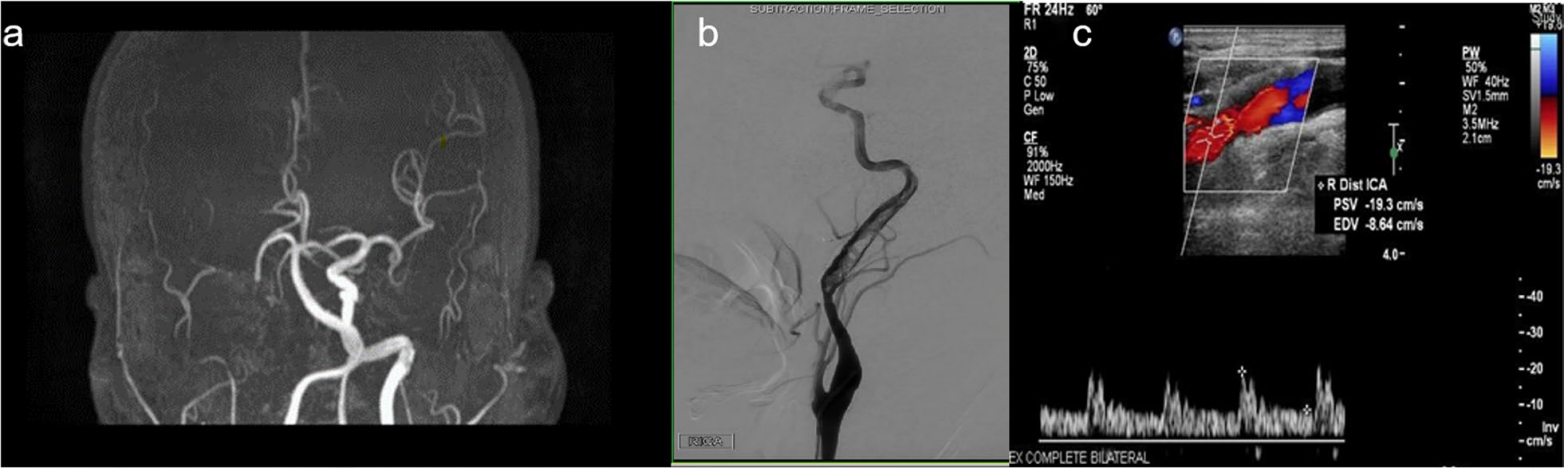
Relevant neuroimaging showcasing key imaging findings. a Right cervicocerebral angiogram with multiple filling defects at the level of the carotid stent just proximal to the petrous bone. Further filling defects extending up through the peak petrous and cavernous segments suggests thrombosis. There is antegrade flow into the middle and anterior cerebral arteries. b Absent flow signal in the right cervical internal carotid artery (ICA) system thought to be pseudo-occlusion. c Right spectral carotid Doppler analysis showing blunting of the arterial waveform consistent with nonhemodynamically significant stenosis of 1–49% in the distal internal carotid artery
